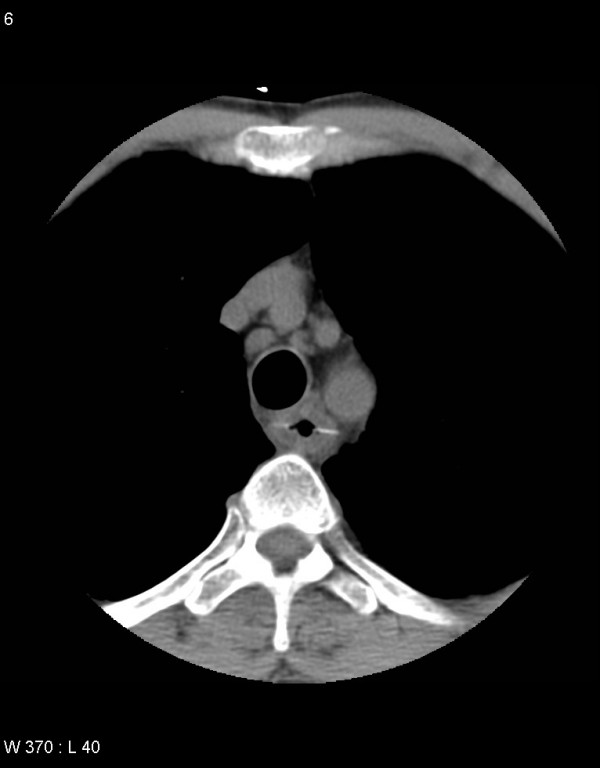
Select axial image of non-contrast phase CT showing a fish bone measuring 3 cm in the oesophagus.
